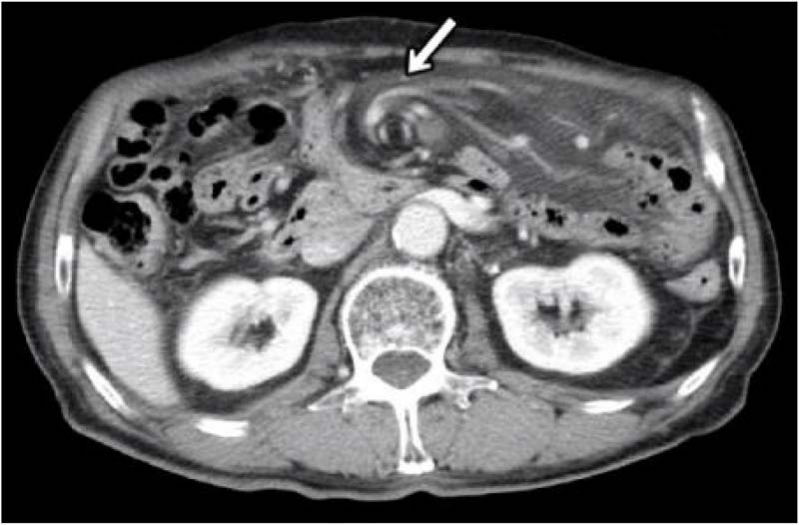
CT at initial diagnosis. CT revealed a whirl sign of the SMA and branches wrapped with the adjacent mesentery and small bowel loops, which are characteristic of SBV (allow).
